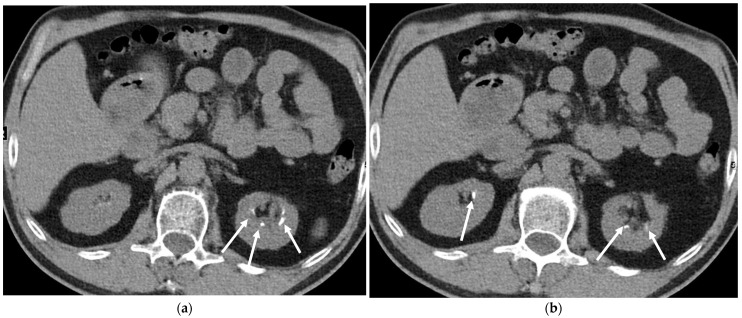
(a,b) Axial low-dose computed tomography scans of a 63-year-old male demonstrating bilateral renal calculi (arrows).
